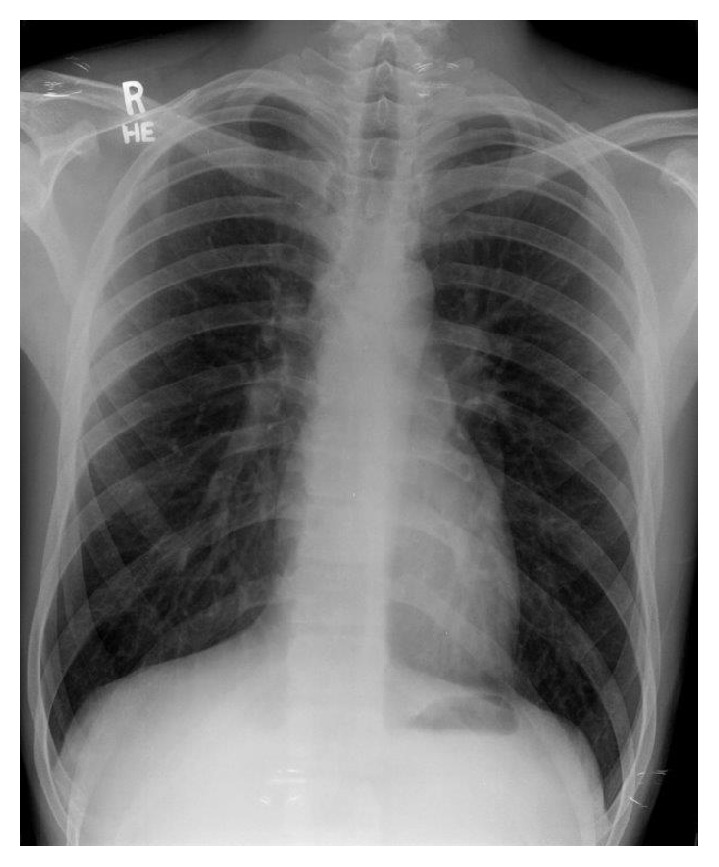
Chest X-ray of patient repeated 1 year later showing complete resolution of the cystic lesions.
